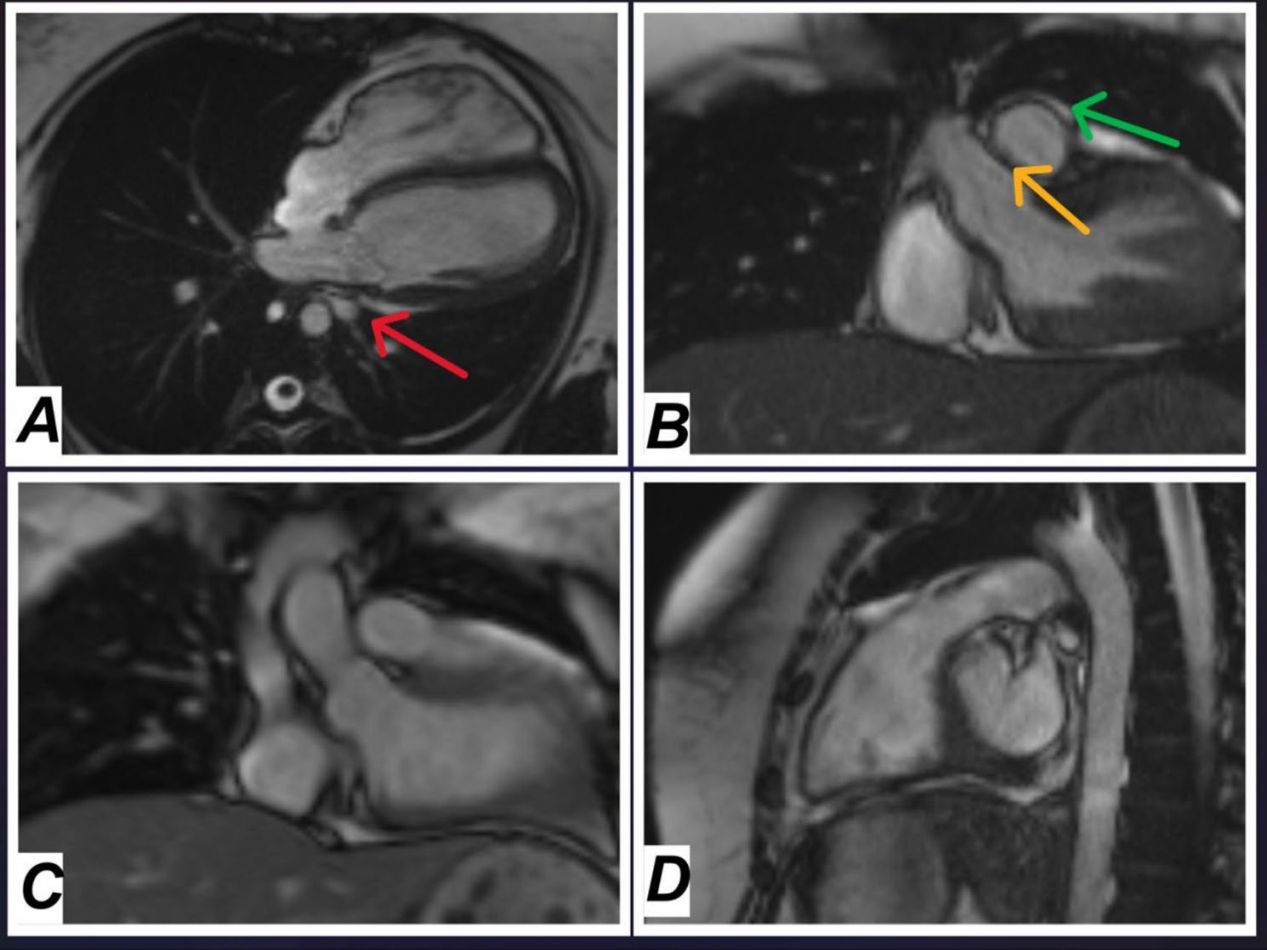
Cardiac MRI confirmed the diagnosis of unilateral agenesis A: Four-chamber view showing narrowing of the caliber of the left inferior pulmonary vein (red arrow) due to compression between the descending aorta and the left atrium, caused by excessive levorotation of the heart secondary to left-sided pericardial agenesis. B and C: Coronal views showing the absence of the left pericardium, along with hyperinflation of the right lung and reduced volume of the left lung. The exaggerated shift of the heart towards the left is evident. The right arrow shows the part of the heart with a pericardium; the yellow one specifies the side where the pericardium terminates. D: Sagittal view demonstrates the left pericardium’s absence, with the heart shifting toward the left hemithorax due to the missing pericardium
